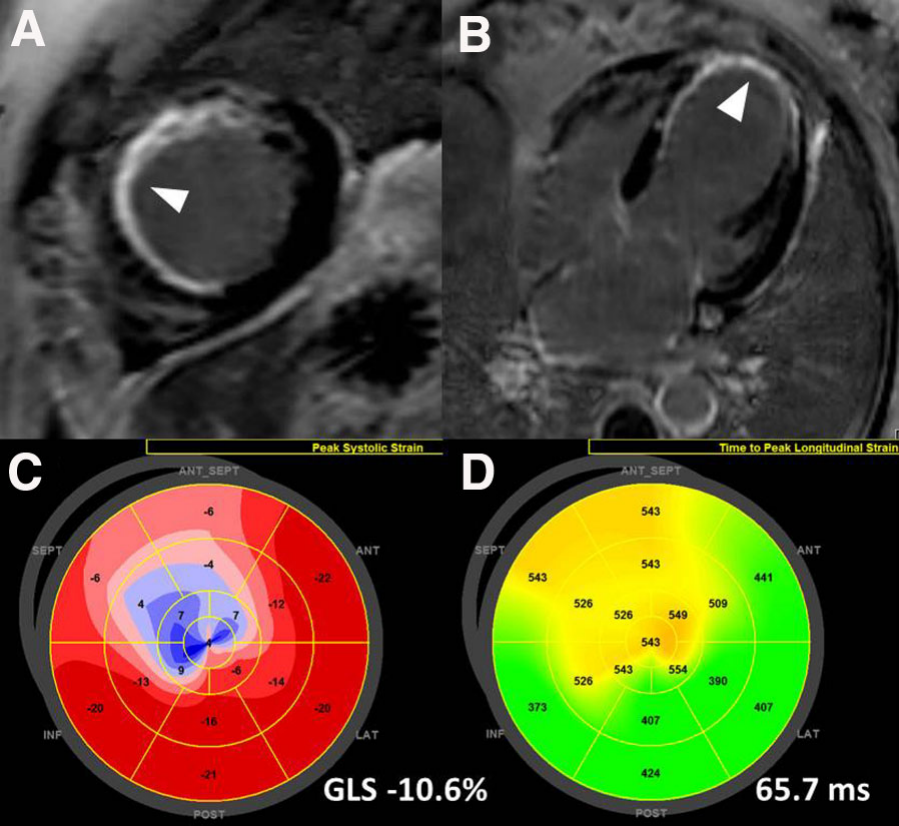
Cardiac magnetic resonance and echocardiographic speckle tracking analysis for risk stratification of patients with ischemic heart disease. Panels A and B show transmural myocardial scar in the apical septal and anteroseptal segments (*arrows*) and subendocardial scar in the mid-inferoseptal segment. On 2-dimensional speckle tracking echocardiography, the magnitude of global longitudinal strain is −10.6% (panel C). The LV apical segments show positive values and are color coded in blue indicating lengthening (correlating with the area of transmural scar). Panel D shows significant mechanical dispersion (65.7 ms) based on the standard deviation of time to peak longitudinal strain of 17 segments. The most delayed areas coincide with the areas with scar and impaired longitudinal strain
